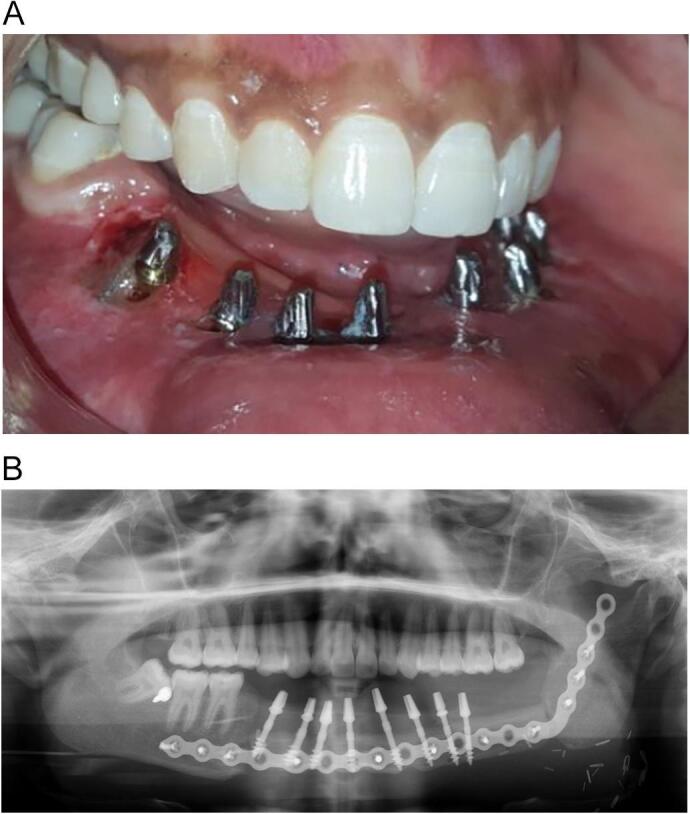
A. An intraoral clinical photograph presenting the distribution of the Corticobasal® implants after impression taken. B. A panoramic radiograph revealing the implant distribution.
